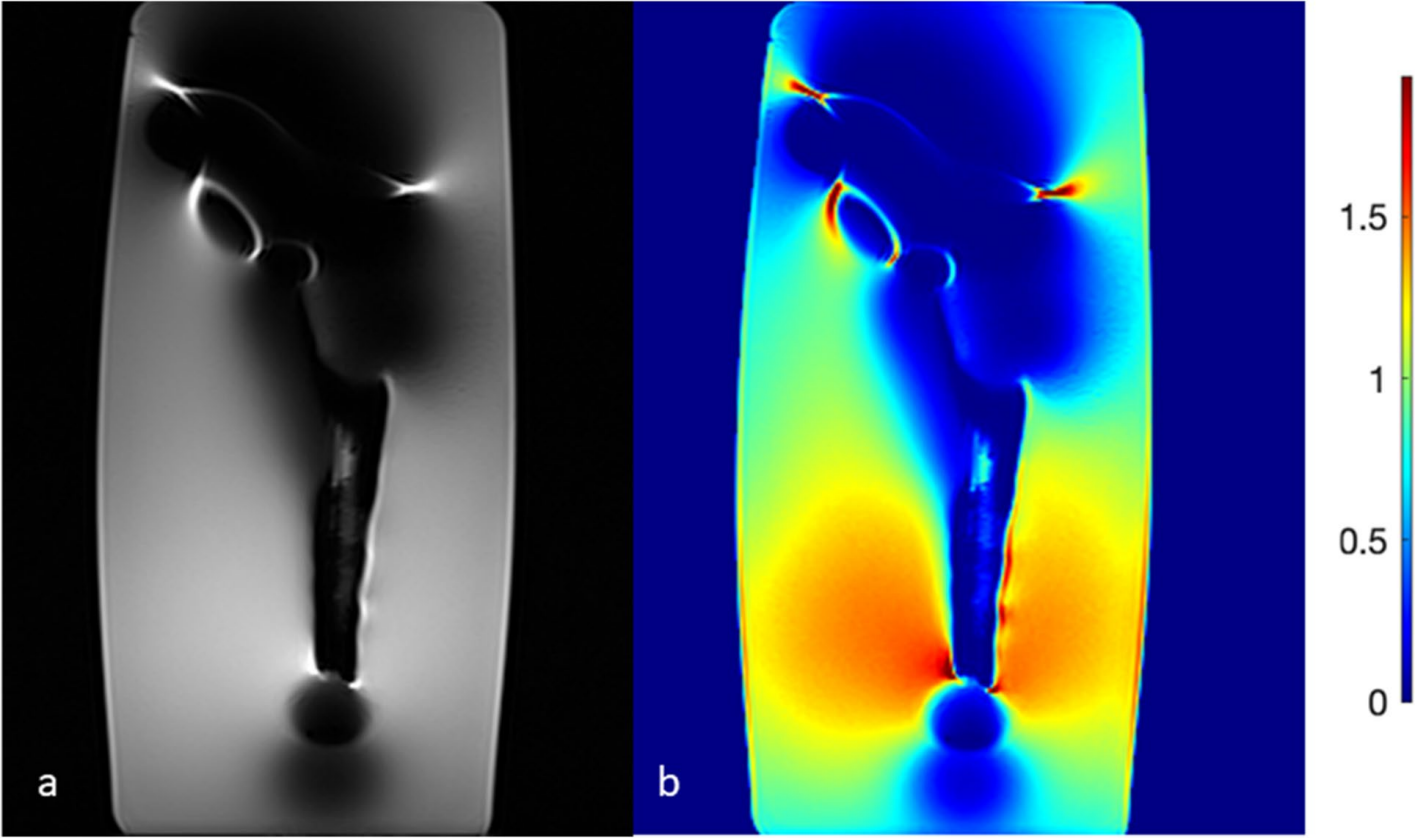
Coronal T2-weighted turbo spin-echo image of an implant (Fe-Cr) at 1.5 T (a) with the normalized signal intensity on the right (b)
